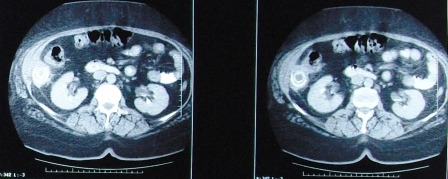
Abdominal CT scan shows a 50-60 mm, concentrically-layered, subhepatic collection with a dense, central, stone-like image, possibly sorrounded by the contrast material injected through the fistula; a segment VI hepatic abcess, exceeding the liver capsule, in direct contact with the walls of the right colonic angle and the ascending colon
